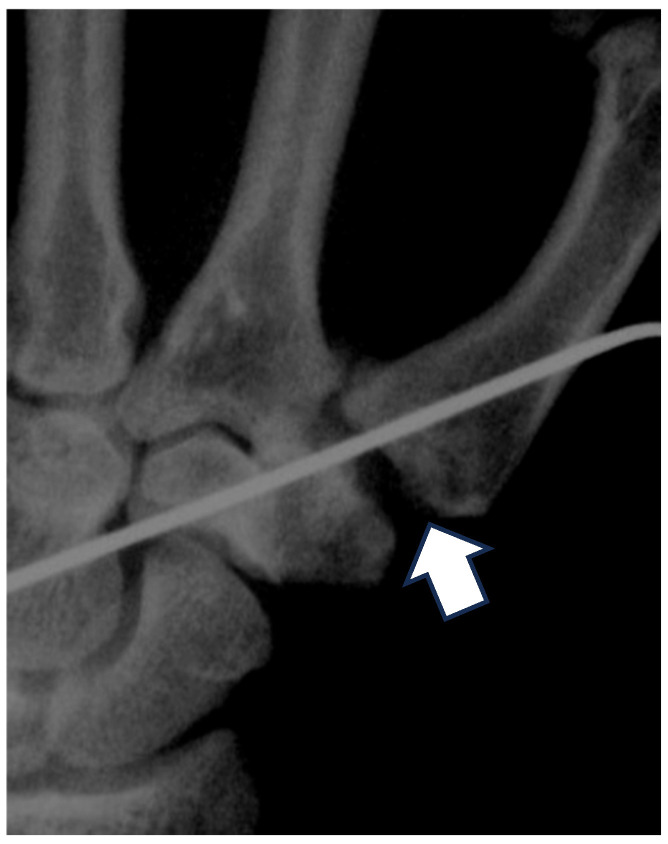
Basal joint fixed using a 1.2 mm K-wire for 4 weeks. Postoperative radiograph at 24-month follow-up showing maintenance of the arthroplasty space. The white arrow indicates the well-reserved space between the base of the first metacarpal and the remaining trapezium.
